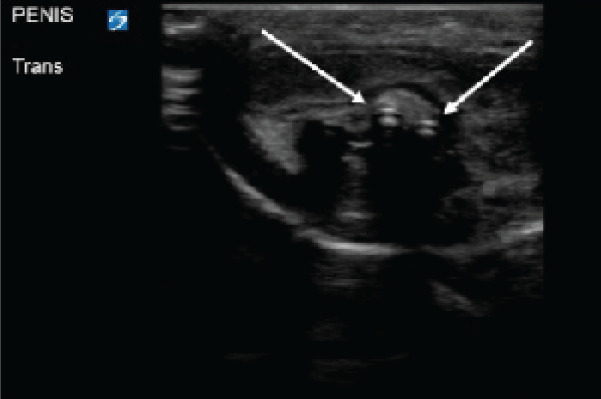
Transverse ultrasound view of the penis using a linear probe. A hyperechoic foreign body (white arrows) seen in the urethra, with evidence of looping of the object.
